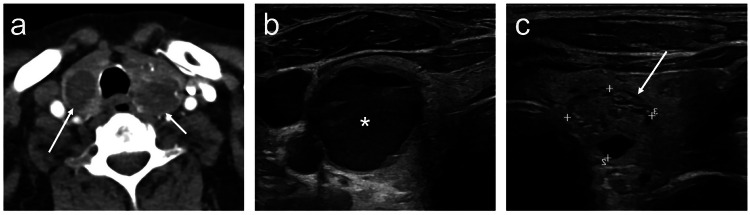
Bilateral ITNs identified on CT (carotid angiogram) (a) in an asymptomatic adult. a CT does not characterise the  thyroid nodules further. b, c On high-resolution US, the nodules’ features of cystic consistency (asterisk), well-defined margins, and spongiform  morphology (arrow) result in a benign grading and no FNA
